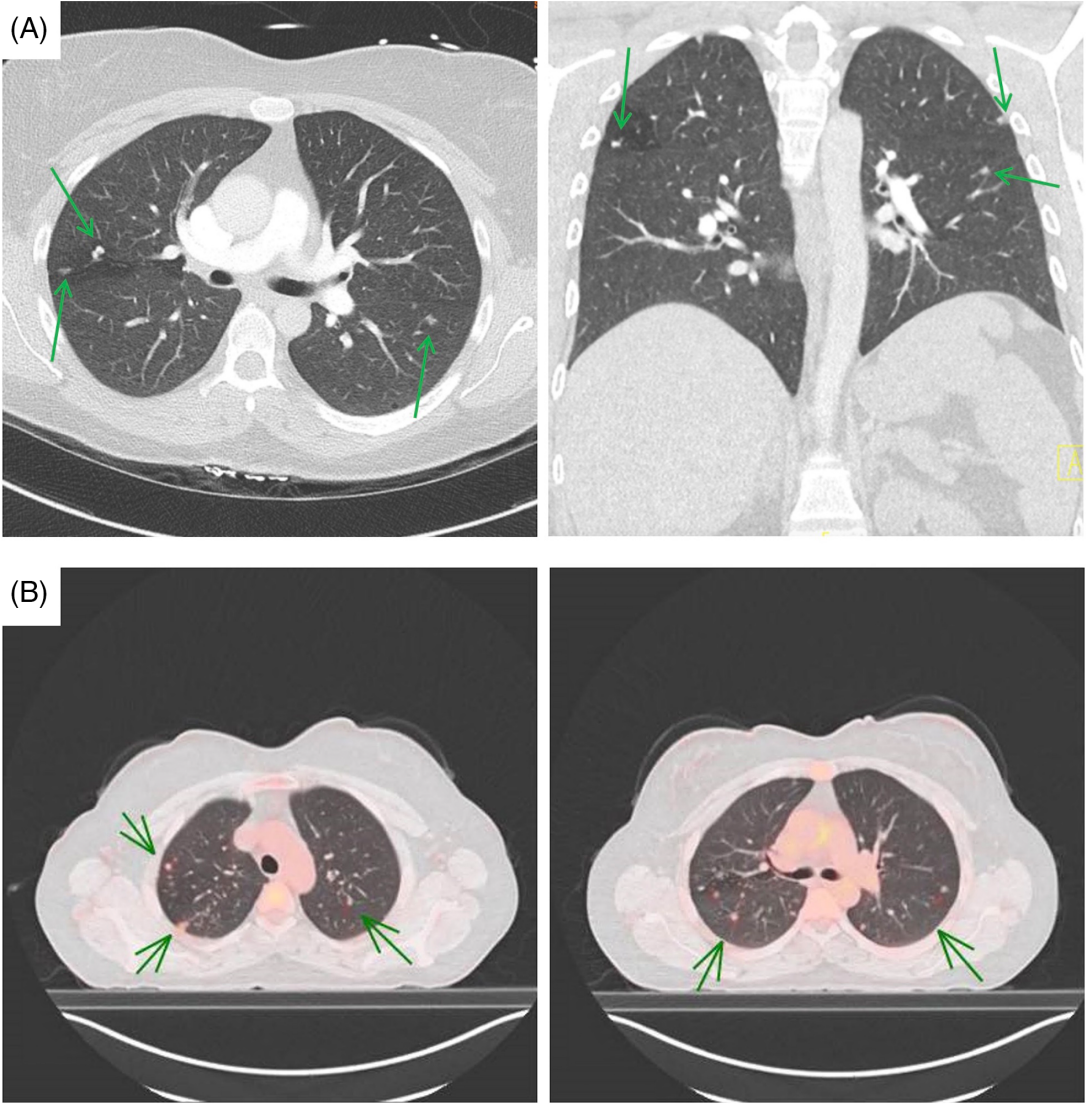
(A) Transverse and coronal slices of initial computed tomography pulmonary angiogram showing multiple bilateral sub‐centimetre nodules in centrilobular distribution. (B) Fused FDG‐PET showing FDG‐avid pulmonary lesions (representative SUV‐max 2.1 subpleural nodule posterior right upper lobe).
